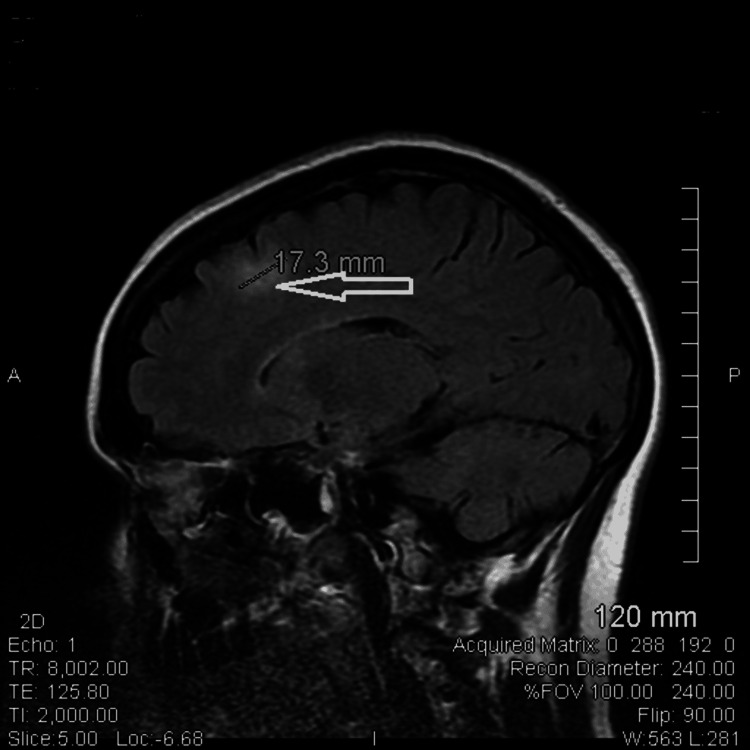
MRI brain with and without contrast shows an enhancing 17.3 mm lesion in the left frontal white matter with surrounding edema.
